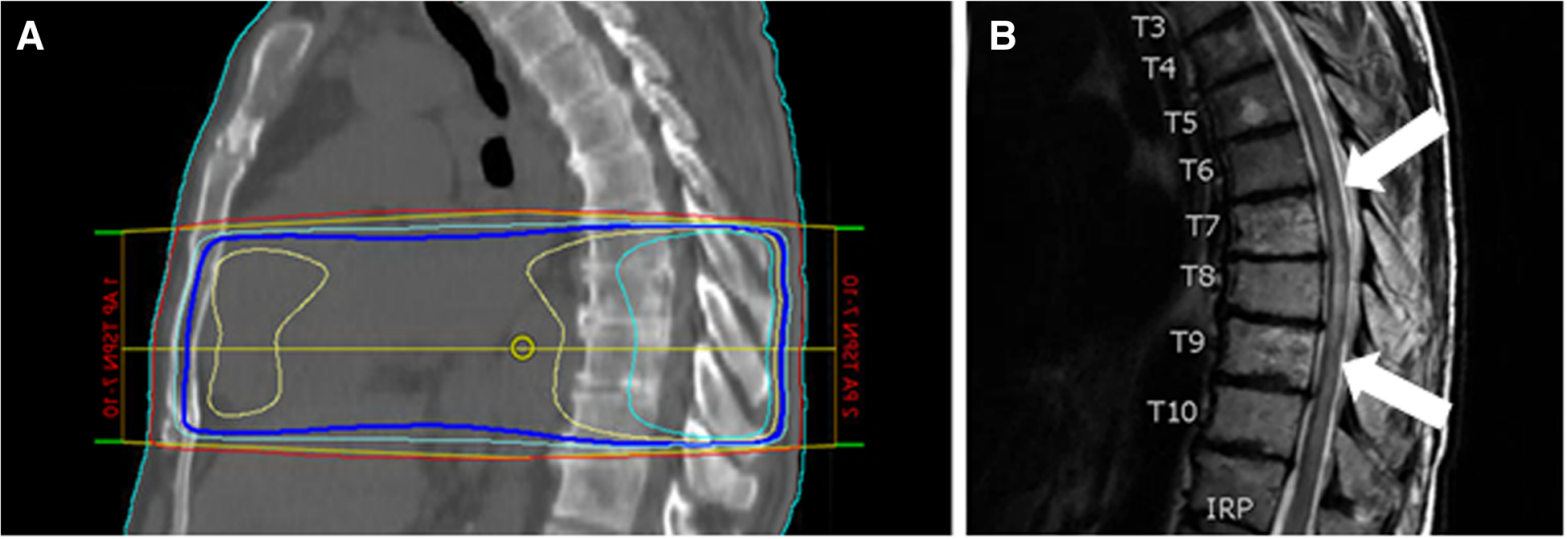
Sagittal images of the (a) radiation treatment fields used for palliative spinal radiation extending from thoracic vertebral level T7-T10 and (b) follow-up spinal MRI obtained 6 months after radiation therapy showing T2 signal abnormality (white arrows) within the region of previous radiation therapy. The dark blue, yellow, and cyan lines in panel A correspond to the 95, 100, 105% isodose lines, respectively
